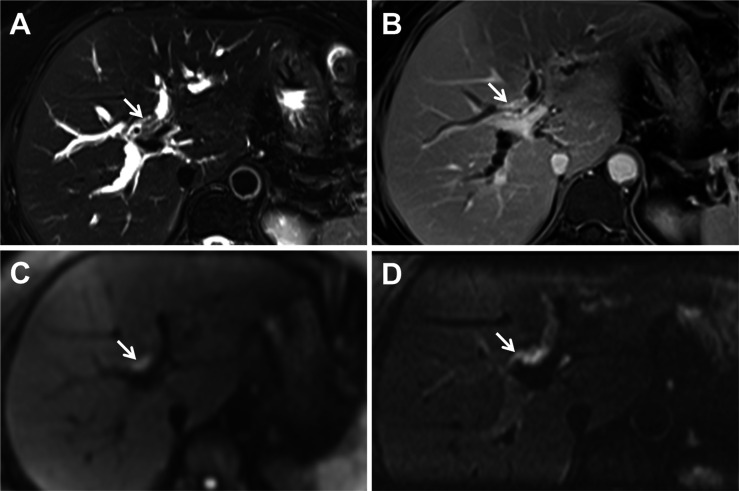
A 54-year-old woman with Bismuth-Corlette type IIIa hilar cholangiocarcinoma. Axial (A) T2-weighted magnetic resonance imaging images indicating the hepatic duct with wall thickening and mild hyperintensities (arrow). (B) Gadoxetic acid-enhanced delay phase image shows thickening of the bile duct at the hepatichilum and the right hepatic ducts (arrow). Conventional echo-planar (c-EPI) diffusion-weighted imaging (DWI) (C) and zoomed echo-planar imaging (z-EPI) DWI (D) were obtained at b=800 s/mm^2^. The lesion bile duct at the hepatic hilum indicated hyperintensities. Both readers interpreted this tumor as type II on the basis of c-EPI DWI (C). However, based on z-EPI DWI, the tumor was clearly observed at the hepatic hilum and the right hepatic ducts were noted as areas of hyperintensity. Both readers interpreted this tumor as type IIIa on z-EPI DWI.
